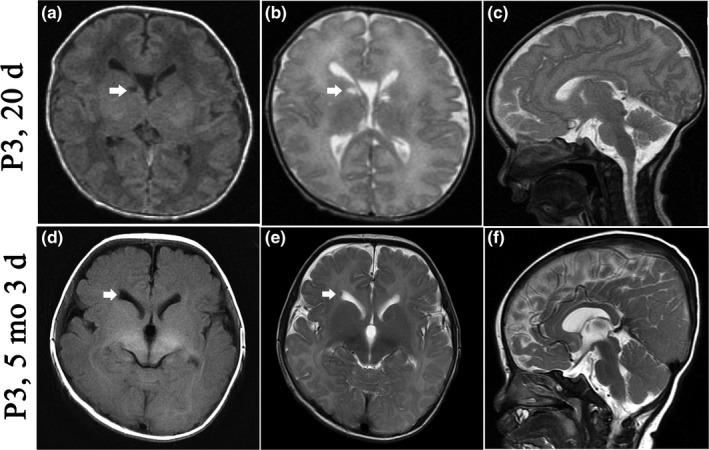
Serial cranial MRI images of Patient 3 with SZT2 mutations. Cystic lesions (white arrow) and widened cavum septum pellucidum were found at 20 day after birth (a, b, c), and the latter was still present at 5 months. Subependymal nodules (white arrow), enlarged ventricle, and shortened corpus callosum were also detected at 5 months (d, e, f)
